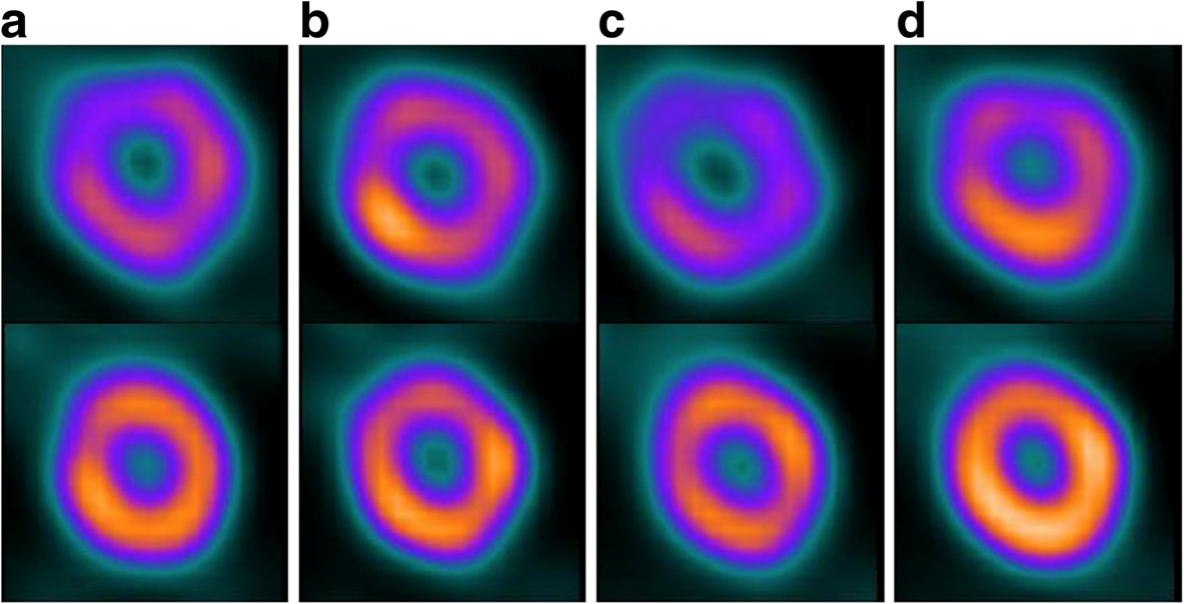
DNM 530c and DSPECT ^99m^Tc and ^123^I uptake. Single ^123^I (a) and single ^99m^Tc (b). Simultaneous ^123^I (c) and ^99m^Tc (d) end-systolic apical short axis uptake for DNM 530c (*upper row*) and DSPECT (*lower row*) for LVEF 50%
